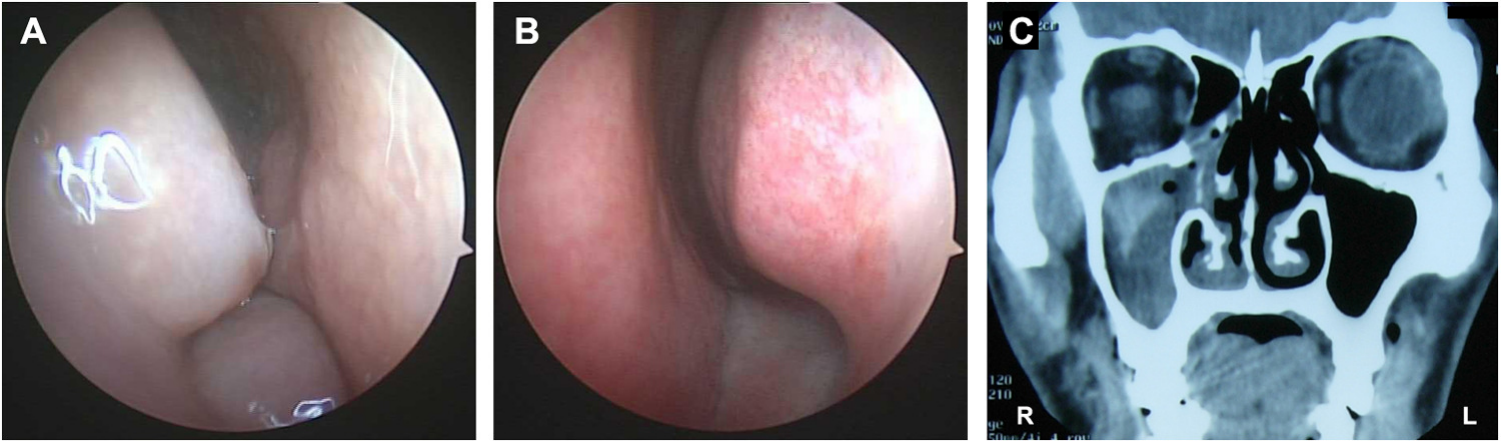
Preoperative nasal endoscopic and sinuses CT finding in AFRS patients. Nasal mucosa on the lesion side was pale and swollen (A), while the contralateral nasal mucosa was normal (B). The affected sinuses were full of irregular, uneven, high-density shadows, which were ground-glass-like, with low peripheral density, and thickened sinus bone (C).
